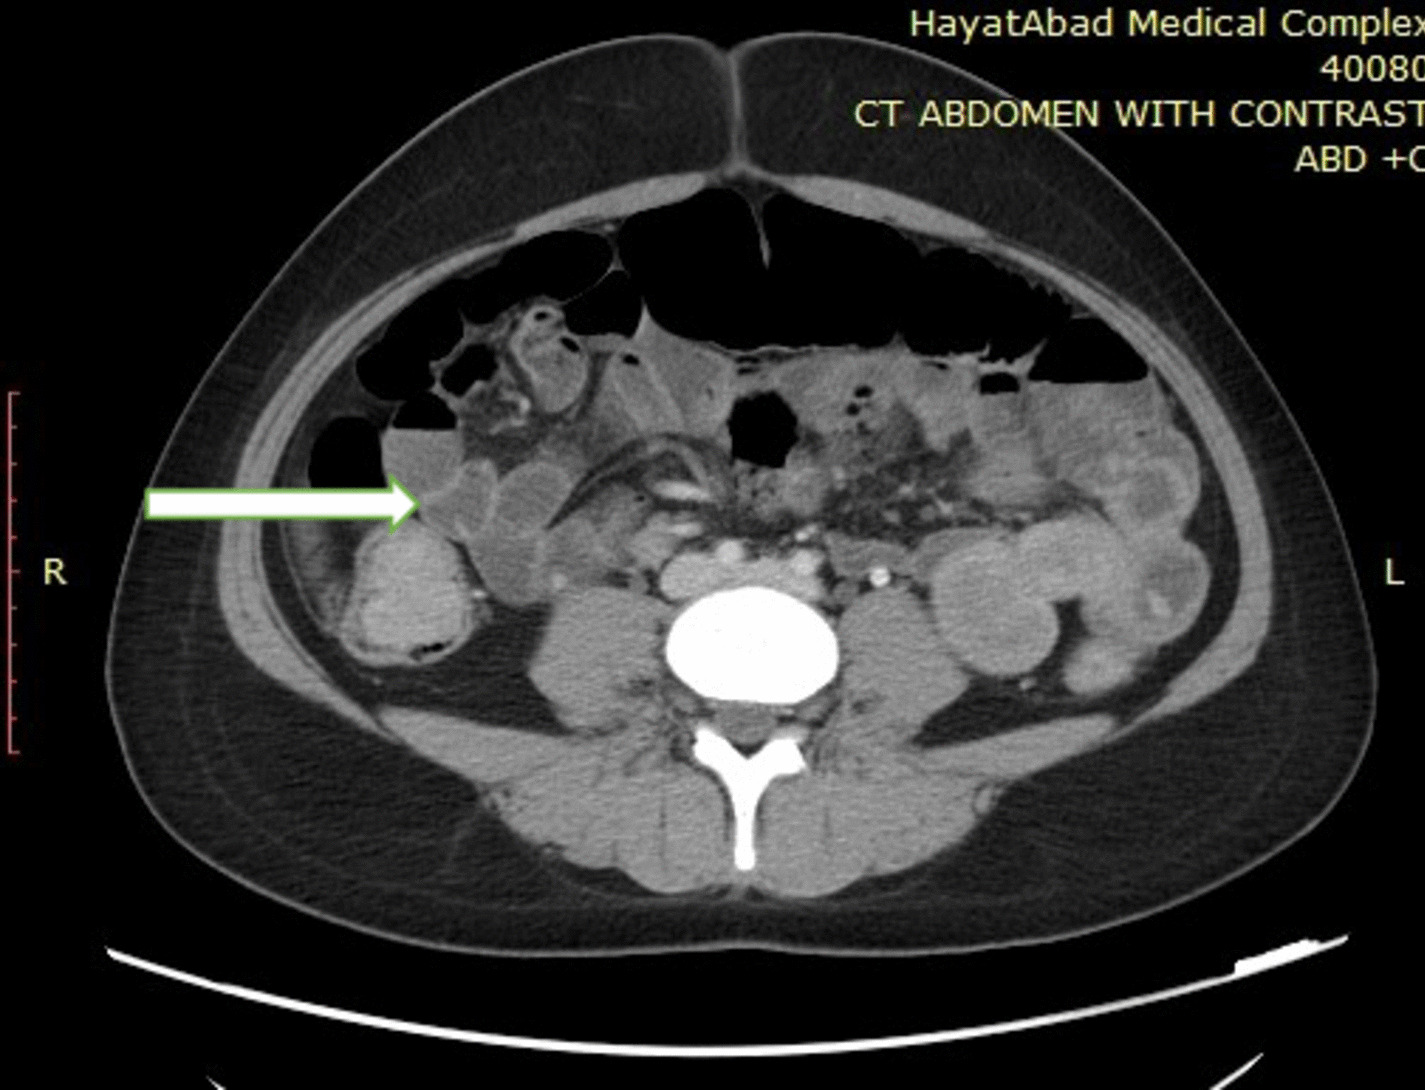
Computed tomography scan with contrast of abdomen and pelvis showing intussusception in small bowel and polyps (arrow)
